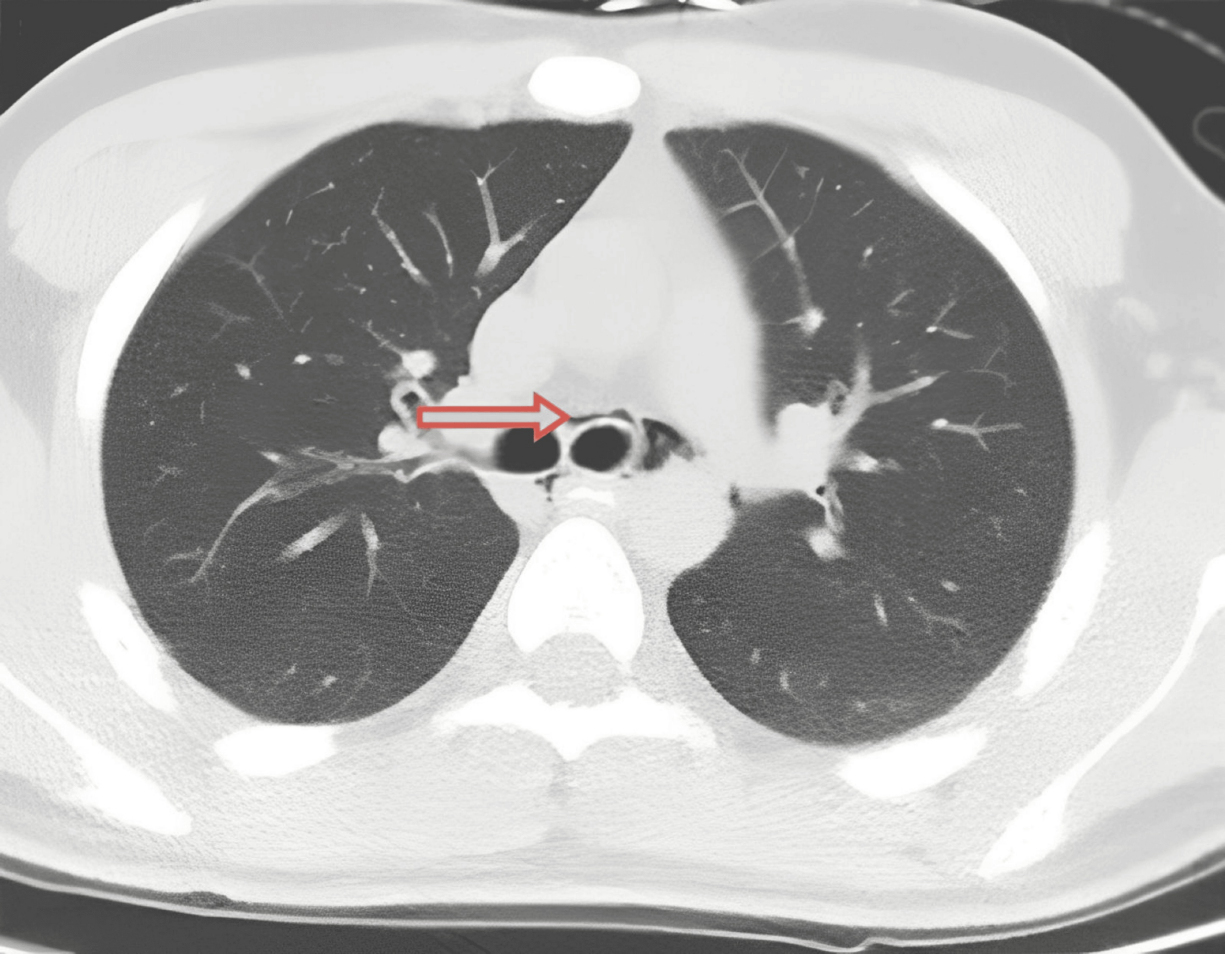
Axial CT image demonstrating pneumomediastinum A thin rim of air (arrow) is visible adjacent to the trachea (the round black airway in the center), dissecting the normally gray-white mediastinal tissue.
